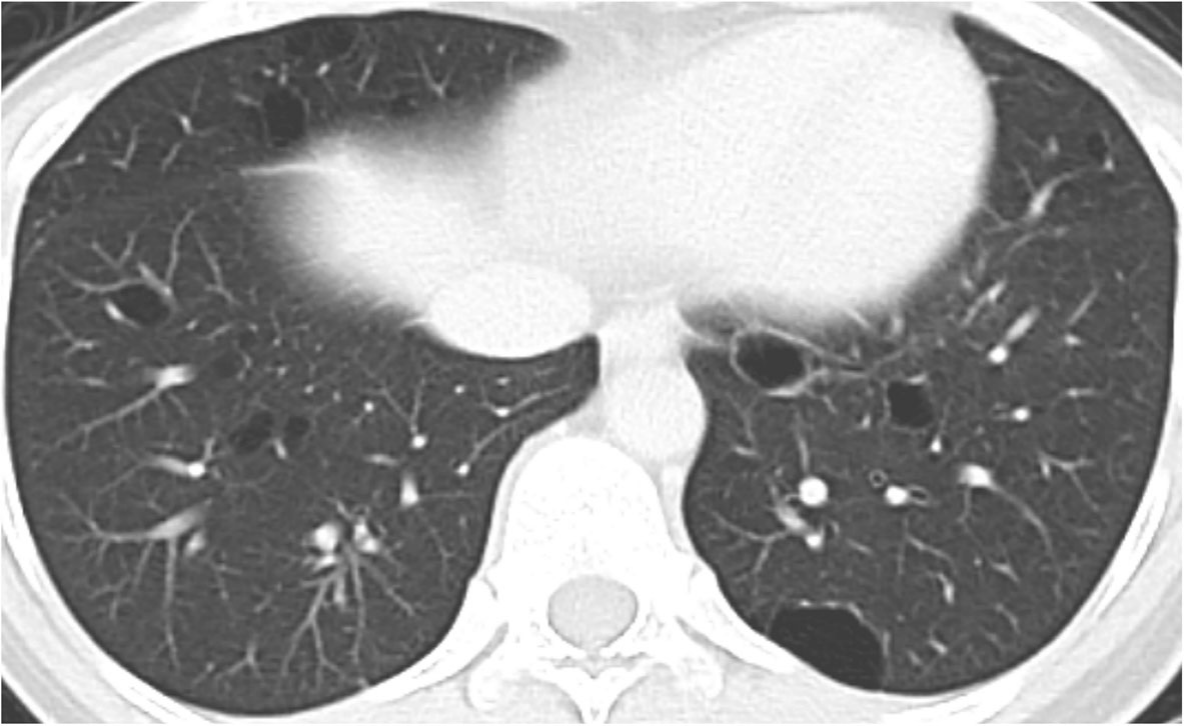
Computed tomographic scan of the chest showing multiple cysts in both lungs
